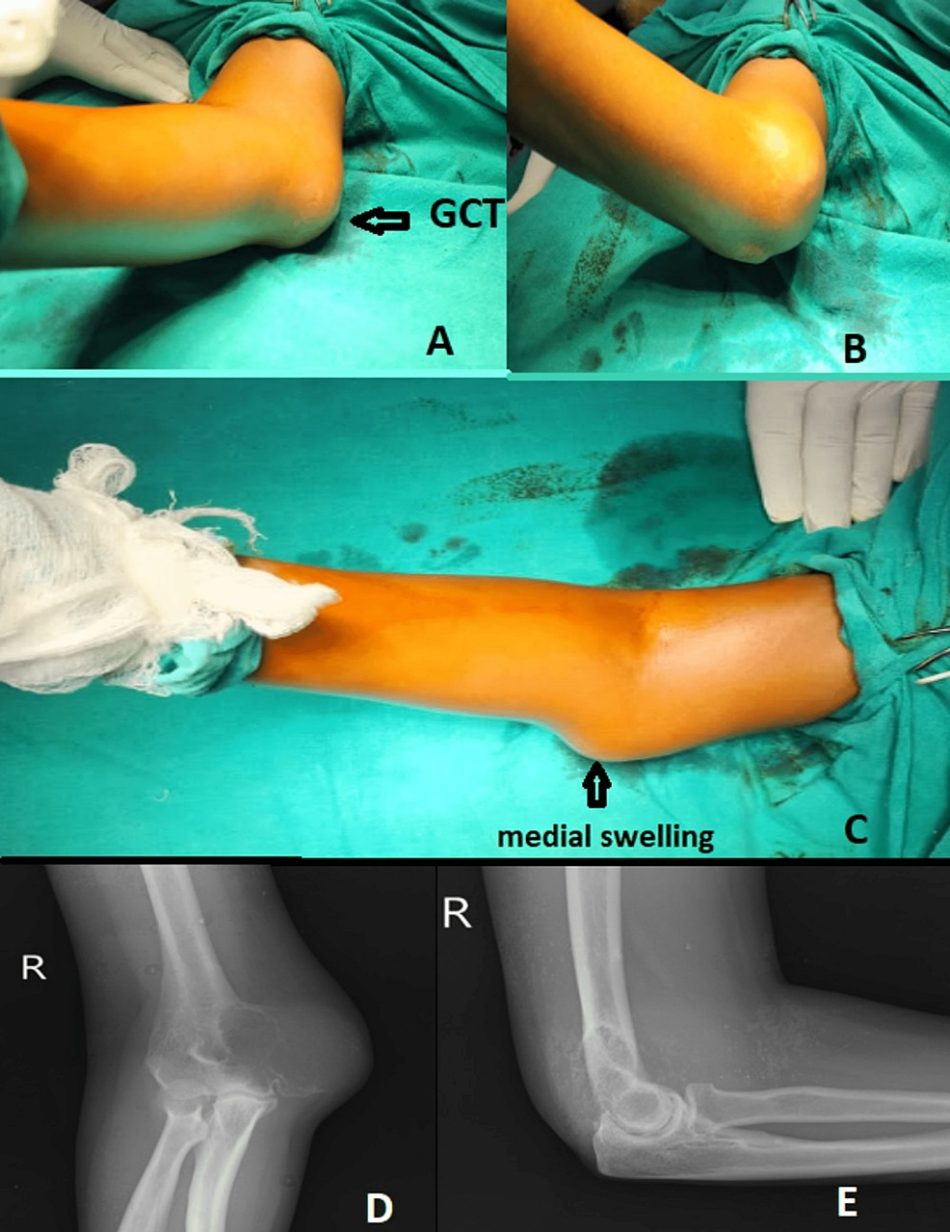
Clinical photographs and X-rays of right elbow in anteroposterior and lateral views showing expansile lesion involving the medial epicondyle of right humerus (A, B, and C) Clinical photographs of the swelling over the right medial aspect of the elbow. (D and E) Anteroposterior and lateral views of the right elbow, showing bony swelling with a soap bubble appearance over the medial epicondyle of the humerus. GCT: giant cell tumour
